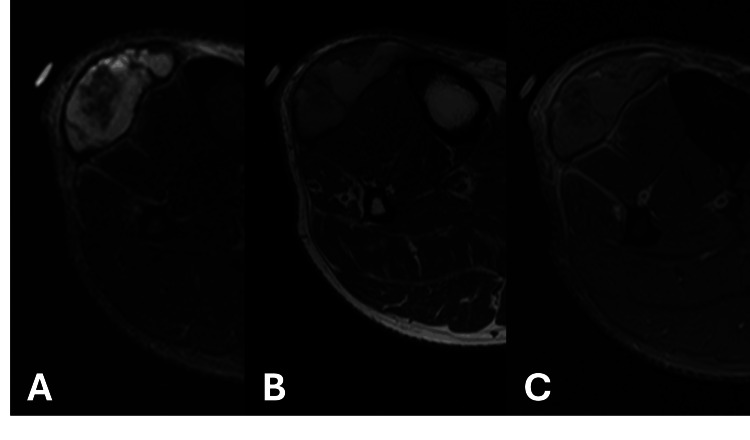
MRI of the right lower extremity with and without contrast A) STIR sequence shows a mixed signal intensity, multilobulated 4.5 × 3.1 × 8.6 cm mass lesion (transverse × anteroposterior × craniocaudal) within the deep subcutaneous fat overlying the extensor/anterior compartment muscles in the proximal leg. There is normal marrow signal of the adjacent tibia; B) There is T1 hyperintensity with thickened, hypointense outer rim; C) Post-contrast T1 demonstrates an enhancing outer wall without internal nodular or thick septal enhancement. STIR: Short T1 inversion recovery
